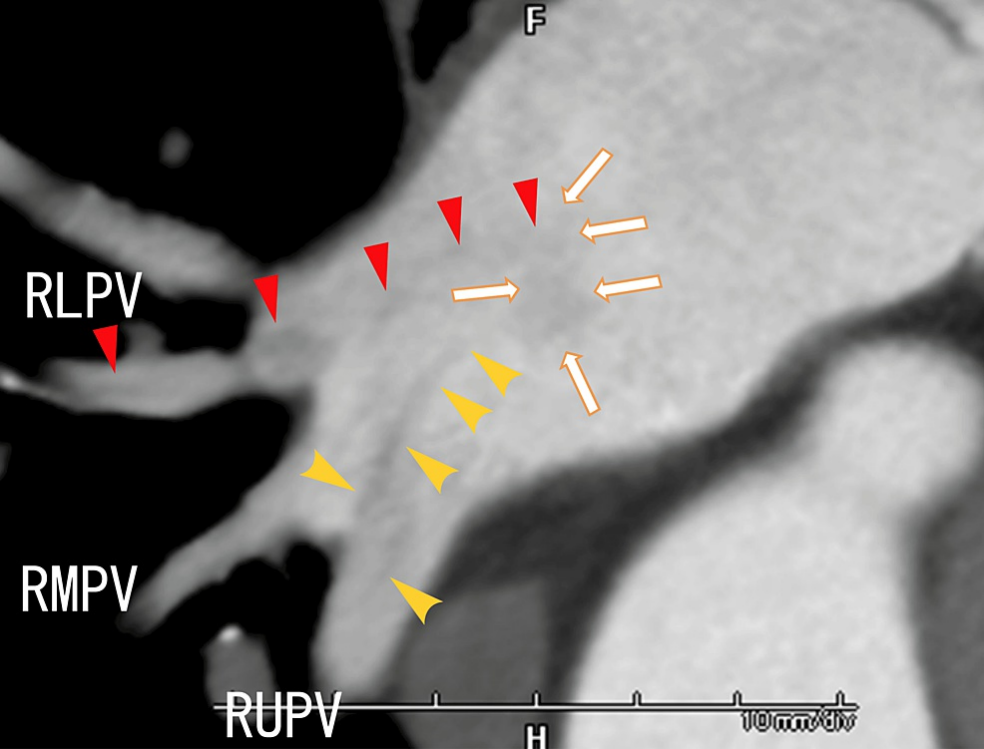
Oblique 80-slice multidetector computed tomography (80-MDCT) images showing thrombi in the right pulmonary veins and the LA. This angle was similar to the angle used for TEE. The yellow arrowheads indicate white LA thrombi extending from the RLPV thrombi, which resemble the white lines depicted using TEE. The red arrowheads indicate the LA thrombi extending from the RUPV thrombi, which resemble white lines with rough surfaces according to TEE. The arrows indicate the attachment areas of both the RUPV thrombi and the RLPV thrombi. There were two right middle pulmonary veins (RMPVs) between the RUPV and RLPV. RLPV, right lower pulmonary vein; RUPV, right upper pulmonary vein; LA, left atrium; TEE, transesophageal echocardiography
